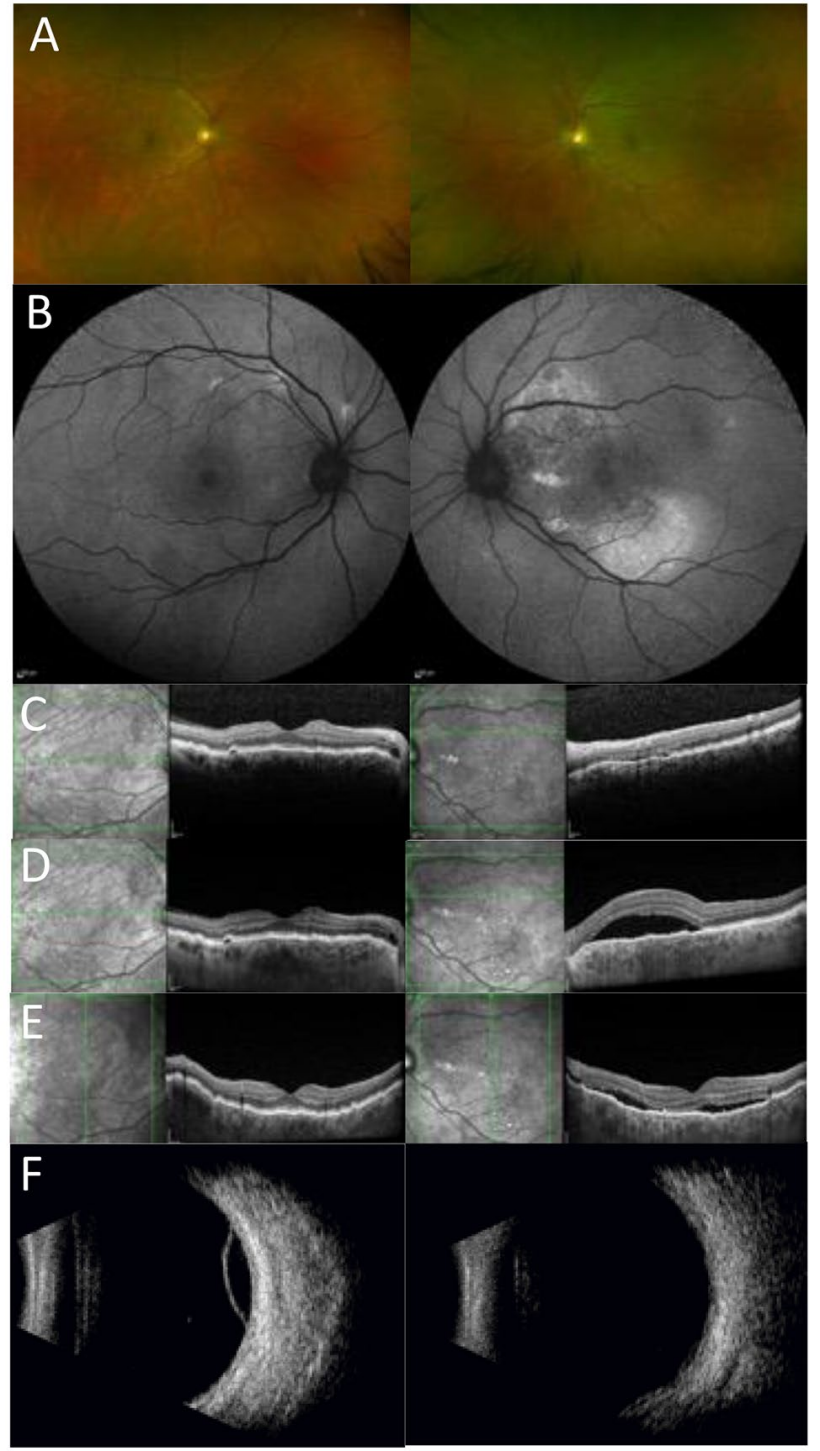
Multi-modal imaging for case 7. (A) Optos imaging showing CRF right eye more distinctly (B) FAF imaging shows bilateral raised autofluorescence signal with significant patches of AF signal increase in the left posterior pole (superior and inferior) with a decreased signal at the left fovea. (C) Baseline OCT images show bilateral CRF, CSC in the left eye, and a small CSC related PED in the right eye with minimal cystic fluid at the right peripapillary region (D) Follow up OCT images again showing the bilateral choroidal folds, significant increase of the CSC neurosensory detachment in the left eye, and stable appearance of the PED in the right eye (E) OCT images with vertical scan showing bilateral choroidal folds and CSC in the left eye at the same time point as in D. (F) Ultrasound B scan showing bilateral thickened ocular coats and peripheral retinoschisis which is more prominent in the right eye
